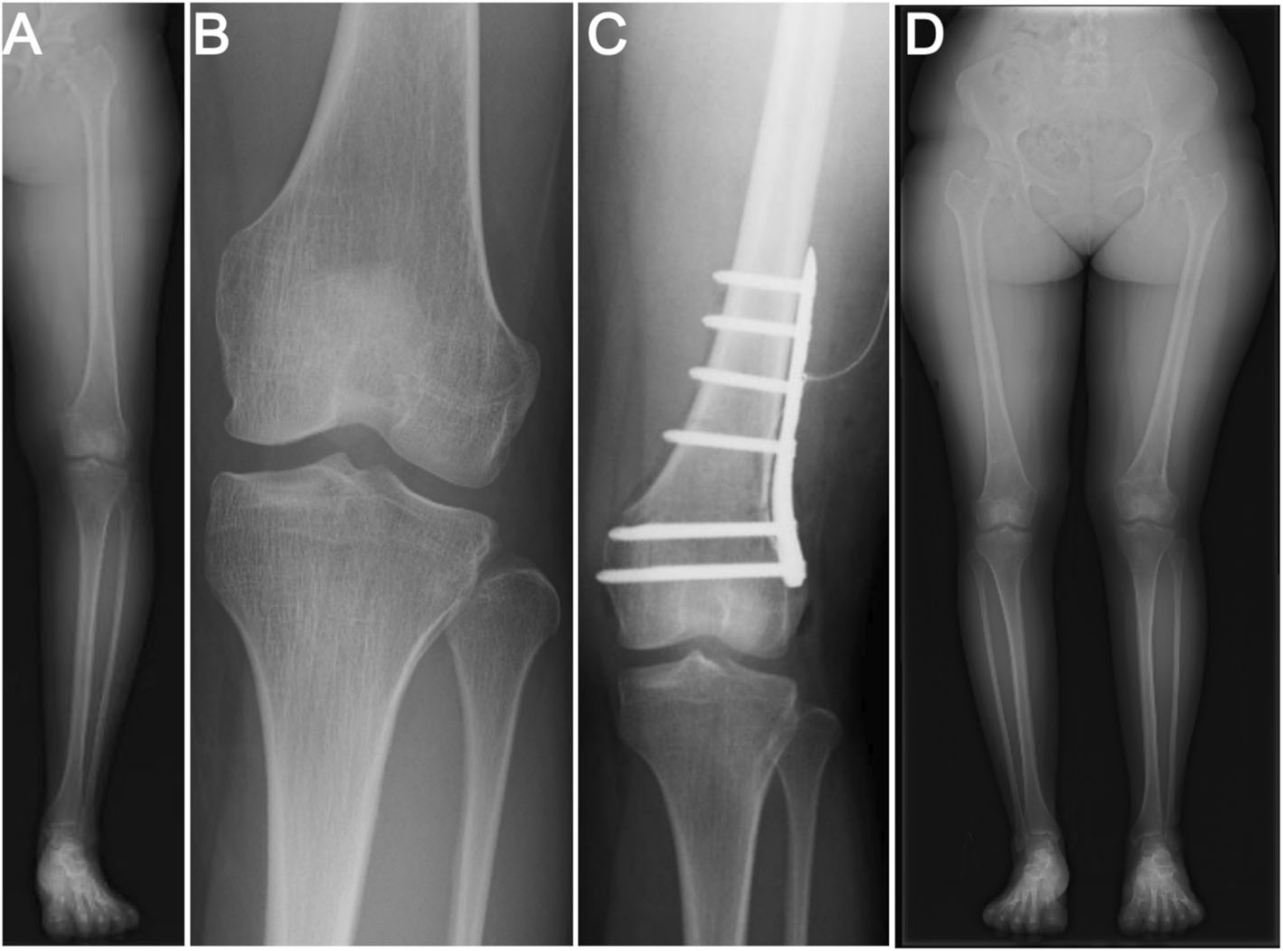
Pre- and postoperative anteroposterior radiographs of the patient by closed wedge osteotomy. a, b Left knee joint showing varus deformity. c Immediately after closed wedge osteotomy. d Final follow-up anteroposterior radiographs of the patient
